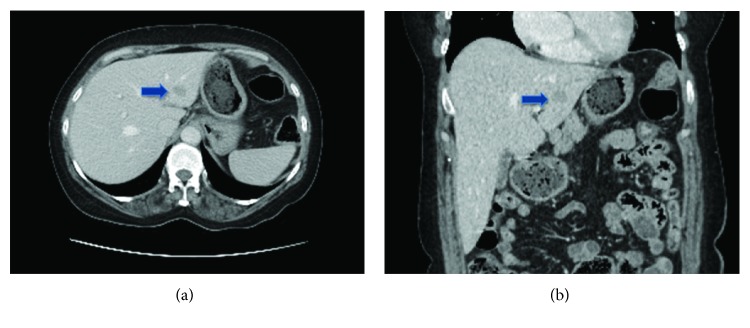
Computed tomography (CT) axial image showing an irregular hypodense lesion in the left lateral section of the liver in (a, blue arrow). The same lesion is shown in the coronal plane in (b, blue arrow).
